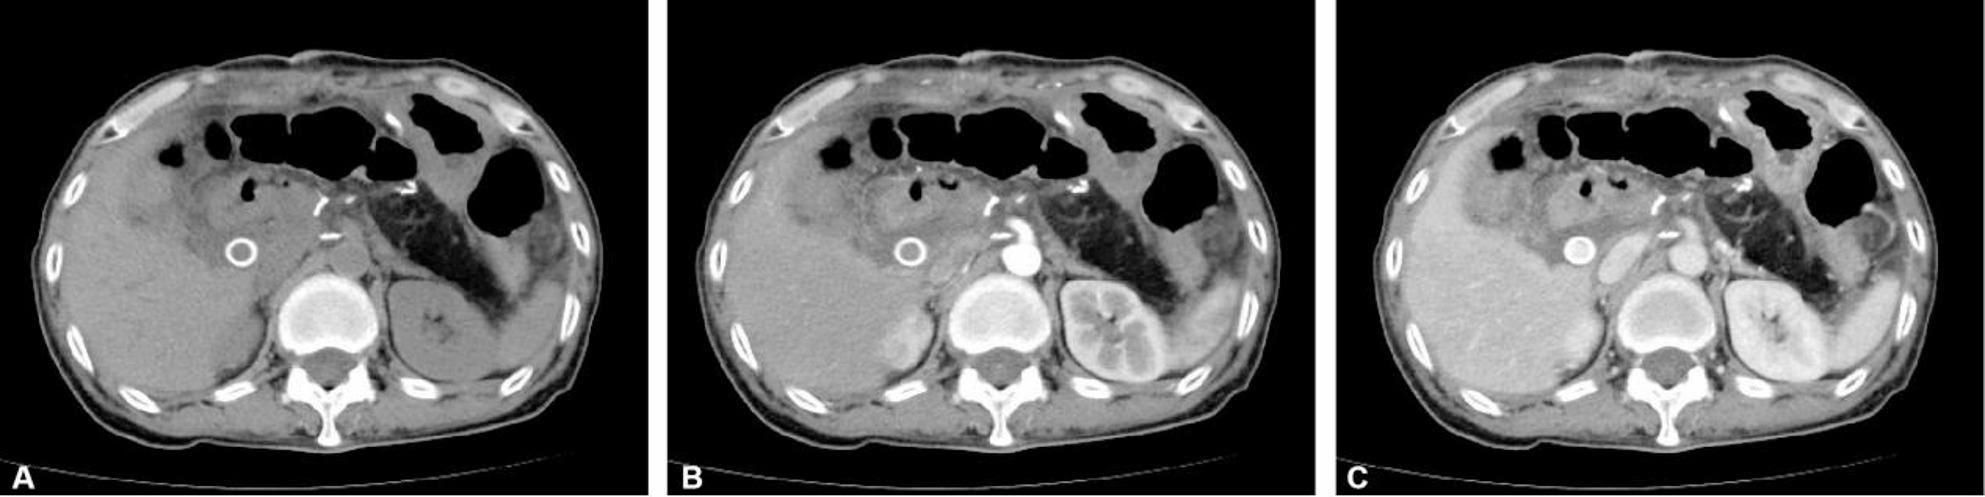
Follow-up CT scan at 1 year postoperatively showing no tumor recurrence, no local fluid collection, patent vascular graft, and preserved hepatic perfusion
